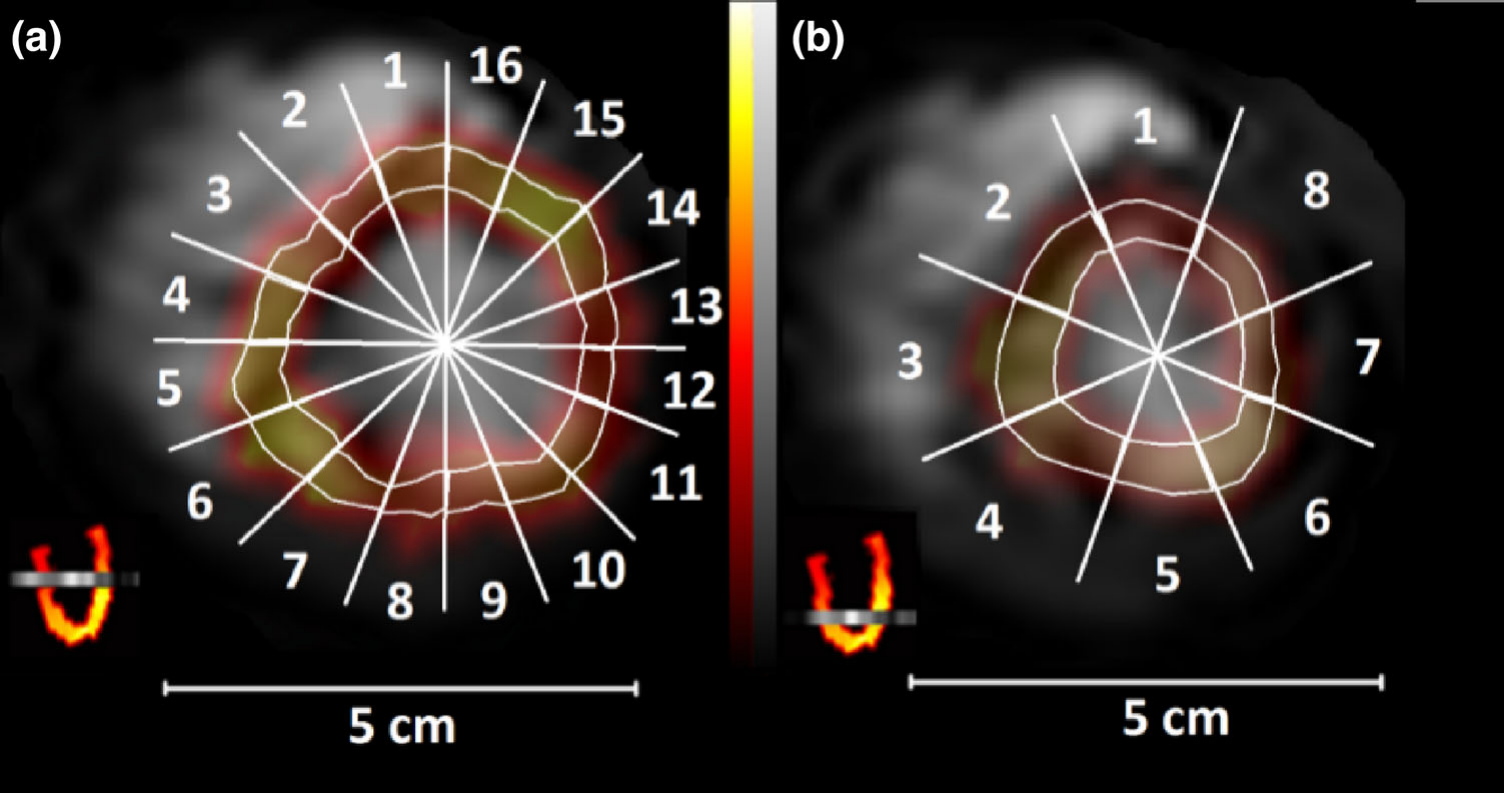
Co-registered short axis MRI- and PET-images with ROIs (delineated with grey lines). (a) A mid-ventricular slice and (b) an apical slice. Orientation of the MRI slices is presented in long axis images (inset in lower left corner). MRI images were acquired using a T1-TFE sequence. Contrast agent can be seen in the right and left ventricles.
